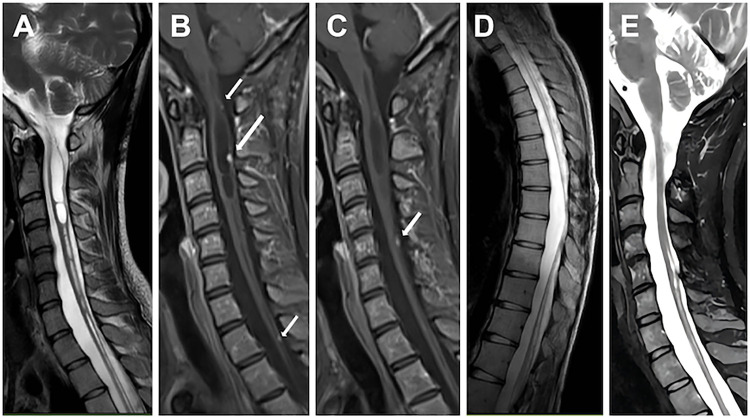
Spinal MRI of Case 2 demonstrating holocord syrinx with multiple intramedullary hemangioblastomas on preoperative imaging (A–D), with contrast-enhanced sequences shown in (B,C). White arrows indicate the distribution of the lesions. Follow-up MRI at 5 years postoperatively showing the evolution of the cervical syrinx (E).
